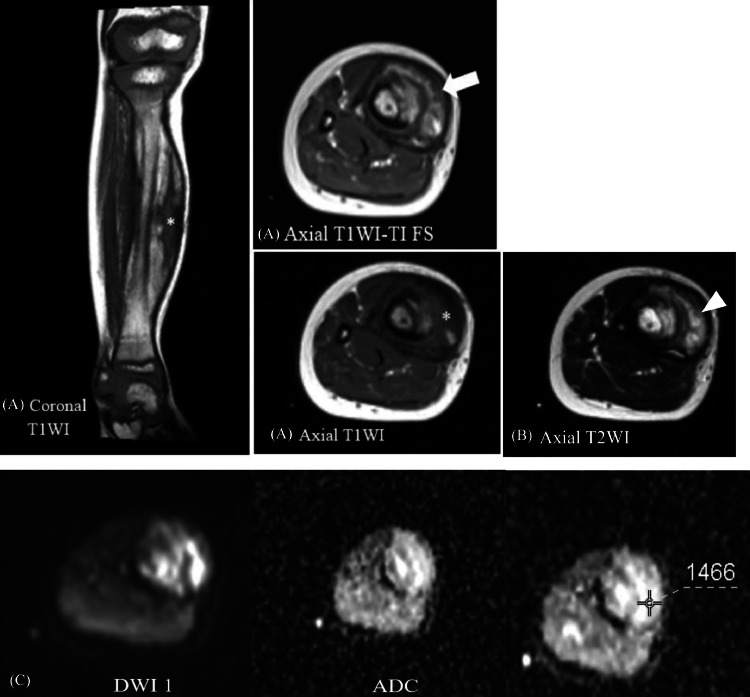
(A) axial and coronal T1WI MRI image show soft tissue mass with a relatively well-defined, irregular-margin, inhomogeneous soft tissue mass (*). The lesion shows inhomogeneous isointense to partially hyperintense signals on T1WI with fat saturation/T1 FS (arrow), (B) heterogeneous hyperintensity on axial T2WI (arrowhead), (C) no restricted diffusion on DWI-ADC (ADC: 1.493 × 10⁻³ mm²/s).
